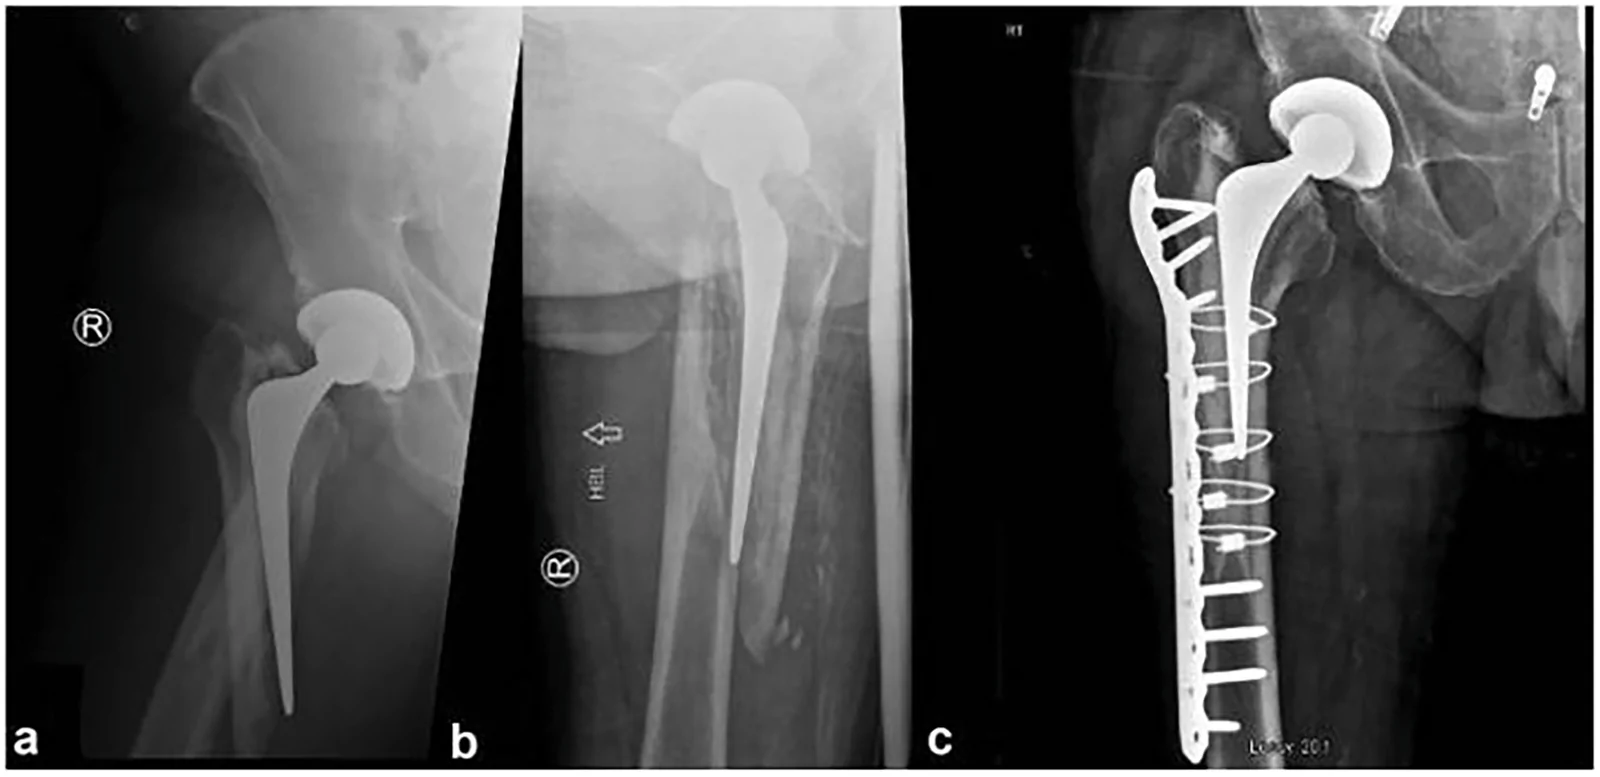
Anteroposterior radiograph of the periprosthetic fracture in a total hip arthroplasty (THA). (b) Vertical orientation of the cross table lateral radiograph. (c) The same patient after cement-in-cement with open reducution internal fixation (ORIF) revision to a short Exeter stem. Adapted from McCarthy et al..^22^
